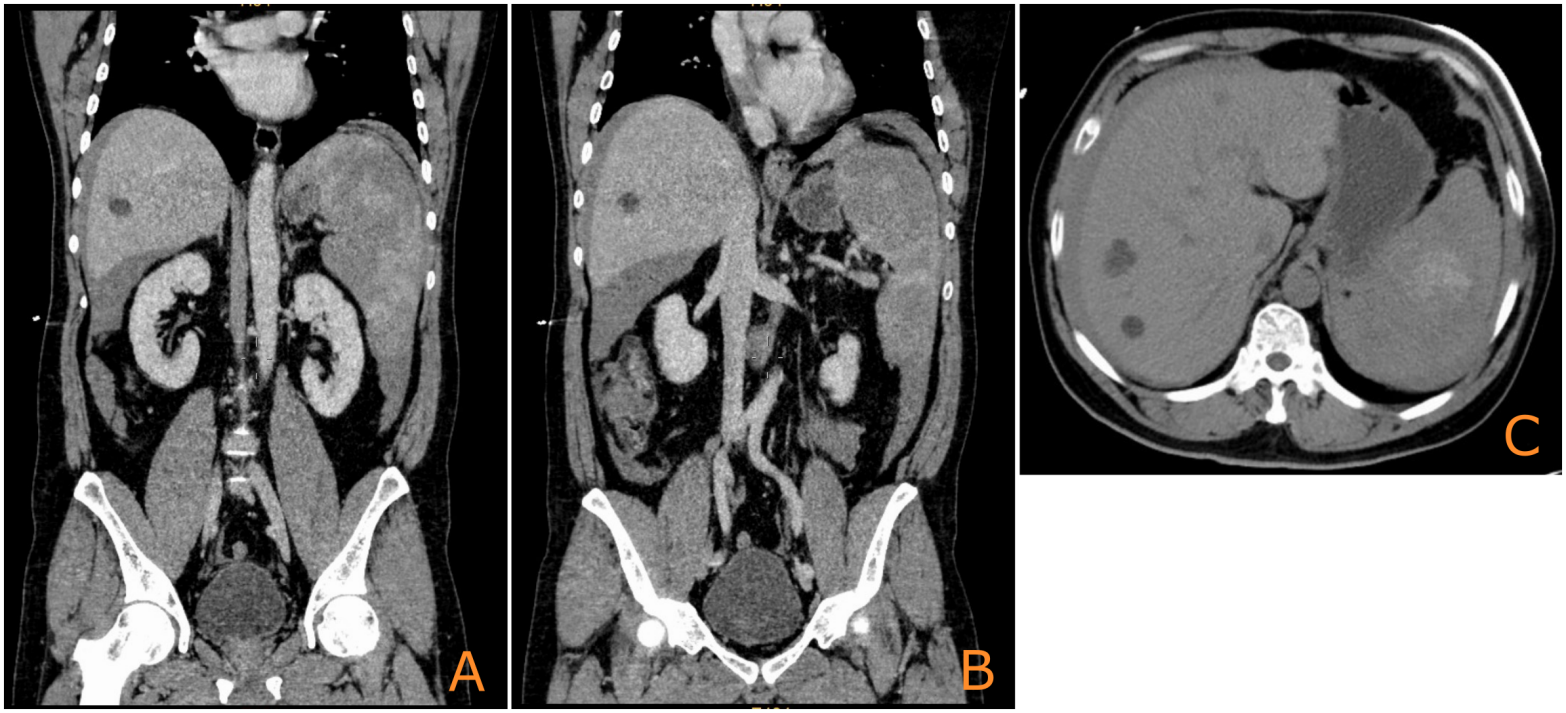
Initial CT image of the spleen upon admission showing multiple nodular occupations [(A, B): coronal plane; (C): axial plane].
